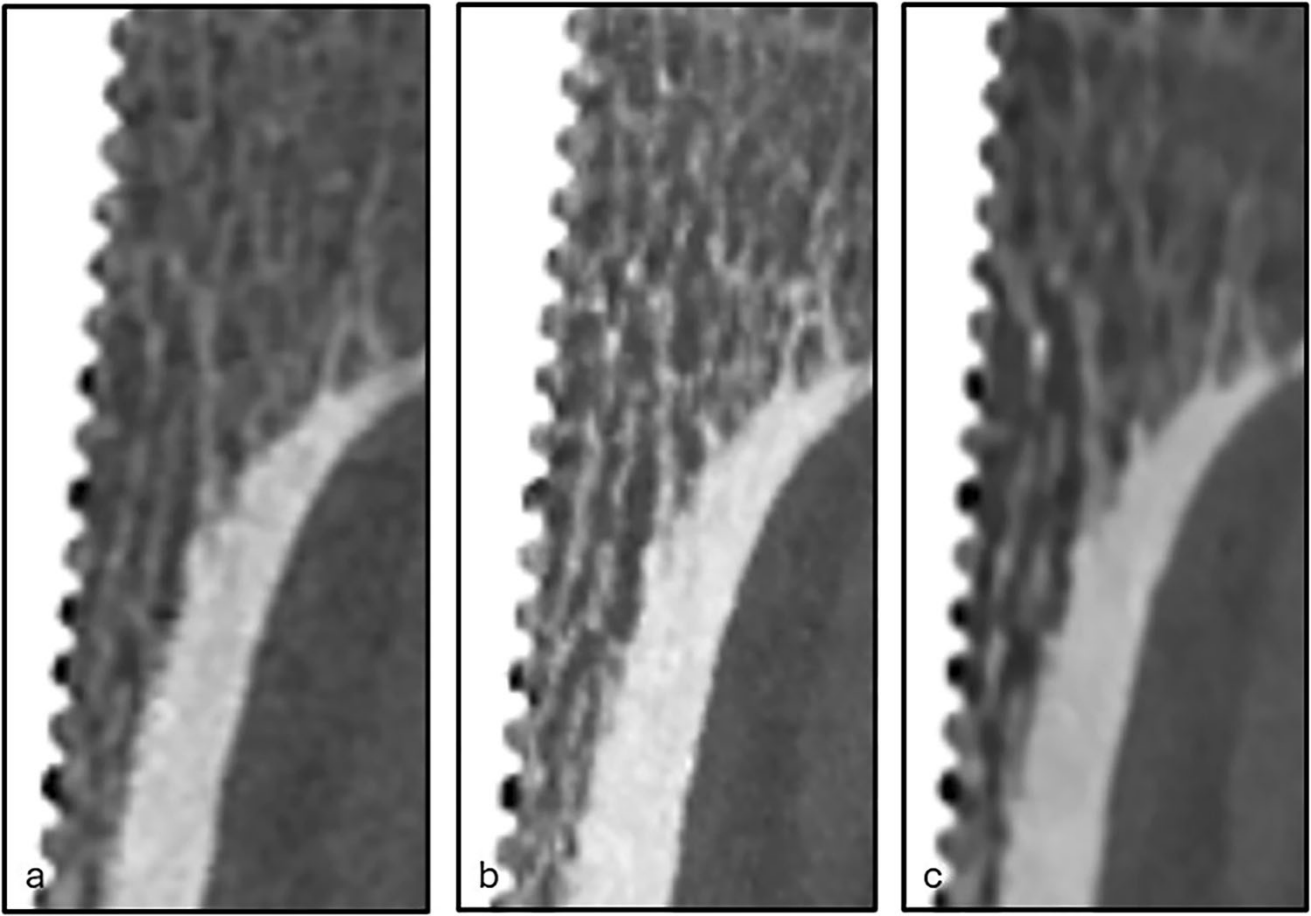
Coronal reconstructions of images obtained using EID-CT (a), PCD-CT polyenergetic images (b), and PCD-CT VMI (c), showing the interface between bone and metal implant. The visibility of trabecular structures was rated highest with PCD-CT polyenergetic images, followed by EID-CT and PCD-VMI. The interpretability of the interface was rated fair or above for all image setups. EID-CT, Energy-integrating detector computed tomography; PCD-CT, Photon-counting detector computed tomography; VMI, Virtual monoenergetic images
